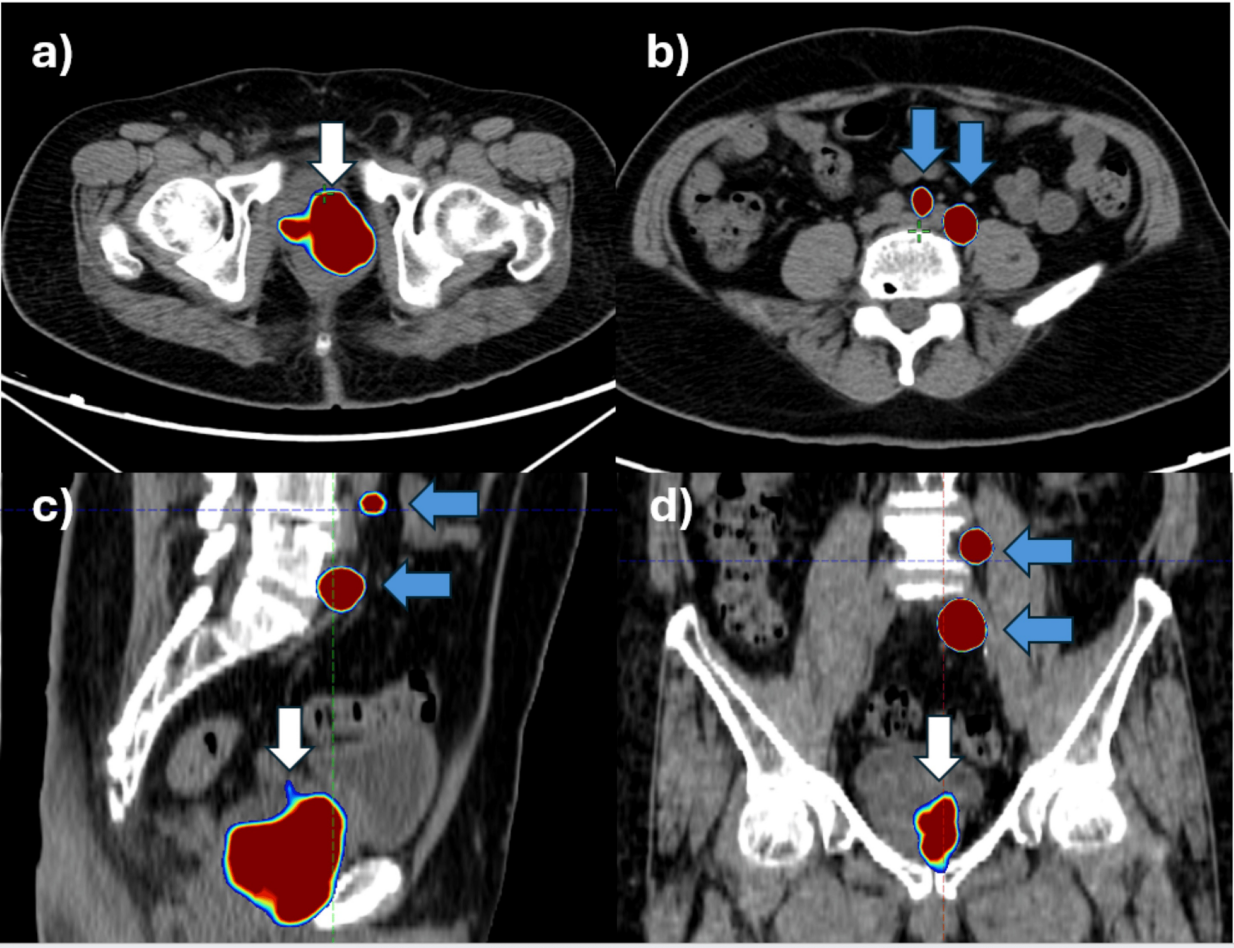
PSMA PETCT shows increased uptake in the prostate (white arrow) and pelvic lymph nodes (blue arrow) in (a) axial, (b) axial, (c) sagittal, and (d) coronal sections PSMA PETCT: prostate-specific membrane antigen positron emission tomography-computed tomography
